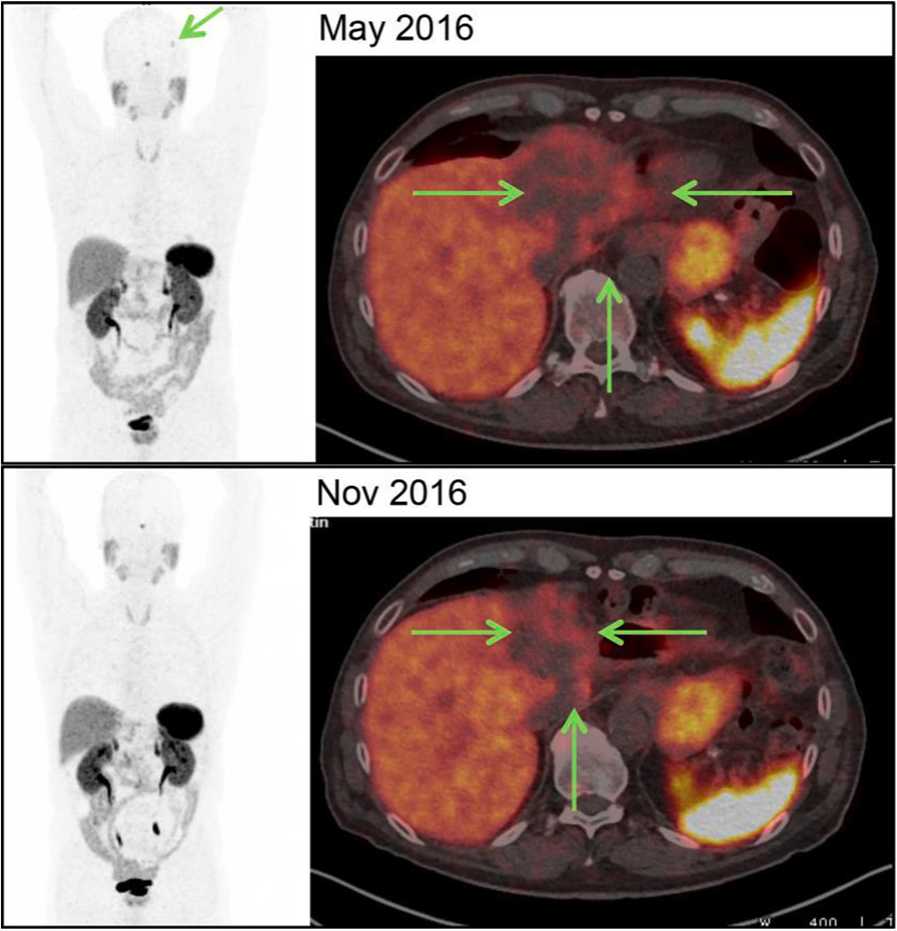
Response to personalized treatment (including olaparib): Ga-68 Dotatate PET/CT. Upper row (May 2016) demonstrating an 8 cm tumor in the left liver lobe below physiologic liver uptake and a SRS-positive brain metastasis. Lower row: (Nov 2016): Follow-up PET/CT with tumor shrinkage to 6 cm and disappearance of the brain metastasis
